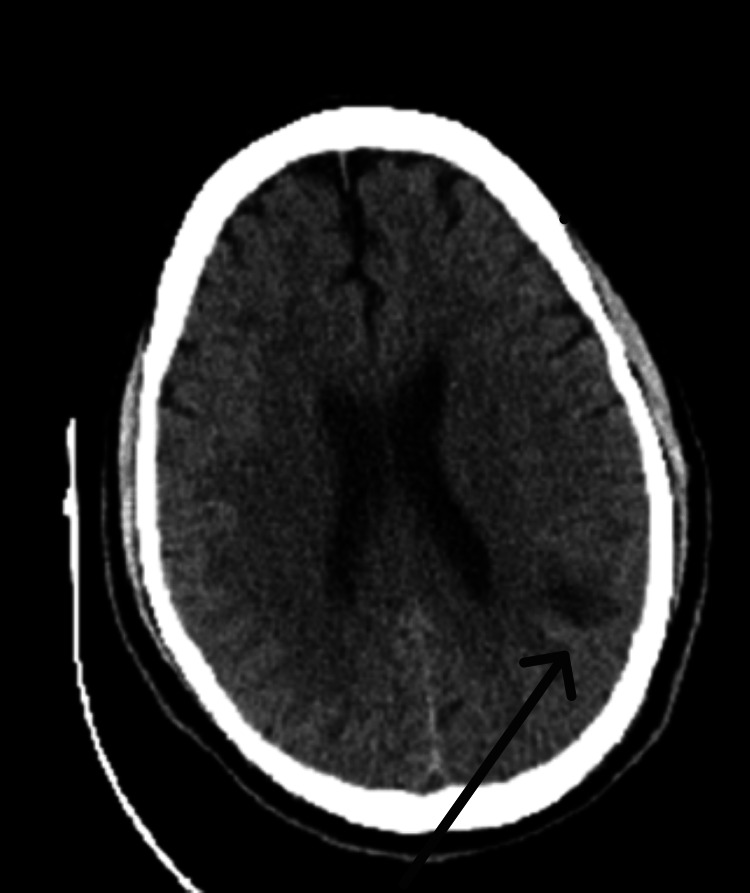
CT head showing ovoid area of hypoattenuation in the left parietal subcortical white matter.
